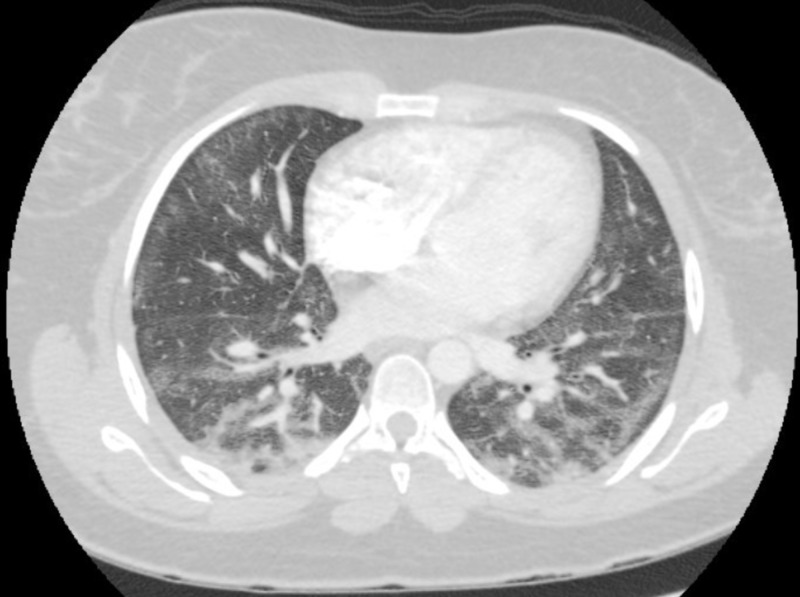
CTA chest showing bilateral ground-glass opacities CTA: Computed tomography angiography
